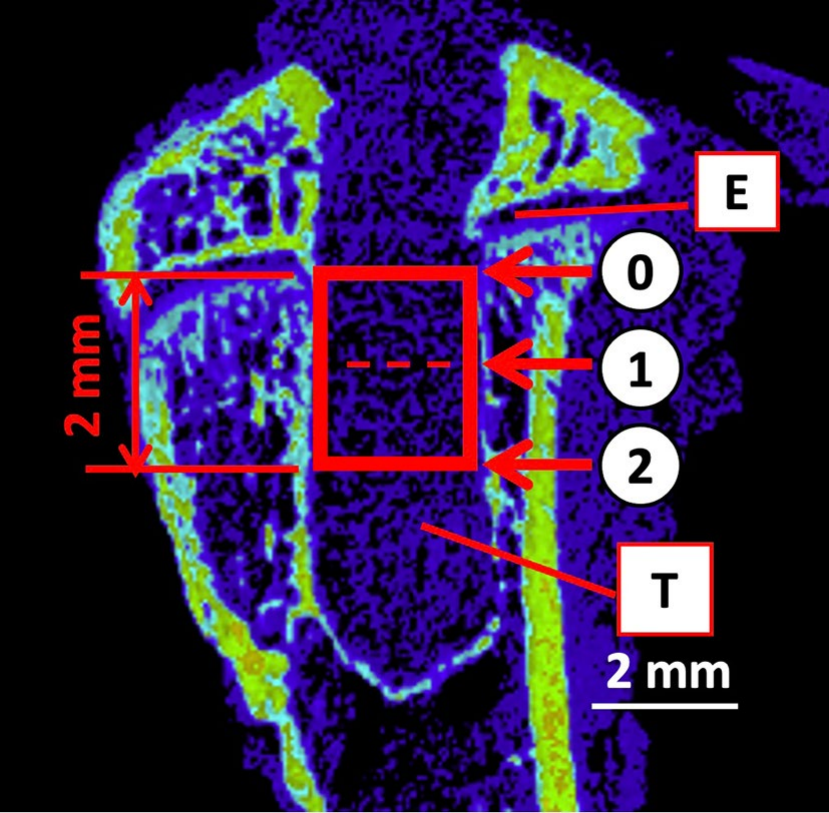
Measurement of bone mineral density at the graft–tibial tunnel interface. The definition of the measurement area of bone mineral density. The CT image shows slices along the long axis of the tibial tunnel. Bone mineral density at the top, middle, and bottom of a region 2 mm distal to the tibial epiphyseal plate was measured. The major and minor tunnel diameters were measured at three cross-sections, namely, the upper (0), middle (1), and lower (2) ends of the region 2 mm distal to the growth cartilage (red square) in the femur–graft–tibia complex. The data on day 0 after anterior cruciate ligament reconstruction were used to determine the baseline diameter of the hole drilled. *CT* computed tomography, *E* epiphysis, *T* tunnel.
